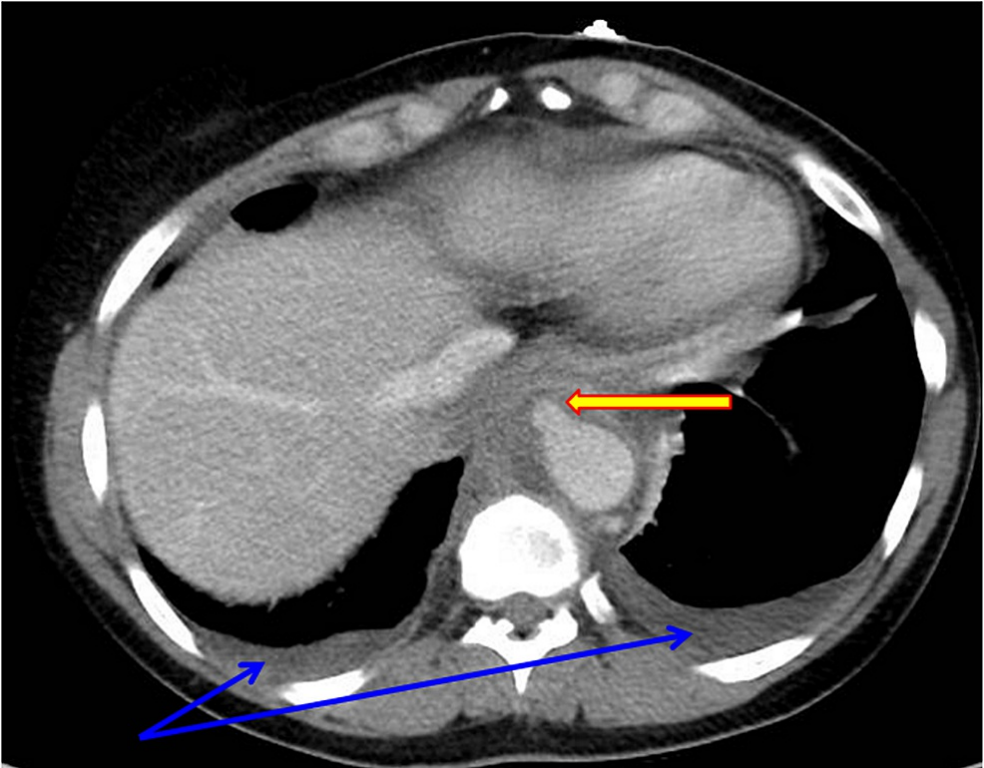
Abdominal CT scan with marked details CT abdomen showing a 1 cm saccular thoracic aortic aneurysm just below the diaphram with adjacent circumferential low density material (yellow arrow) and bilateral mild pleural effusions (blue arrows)
